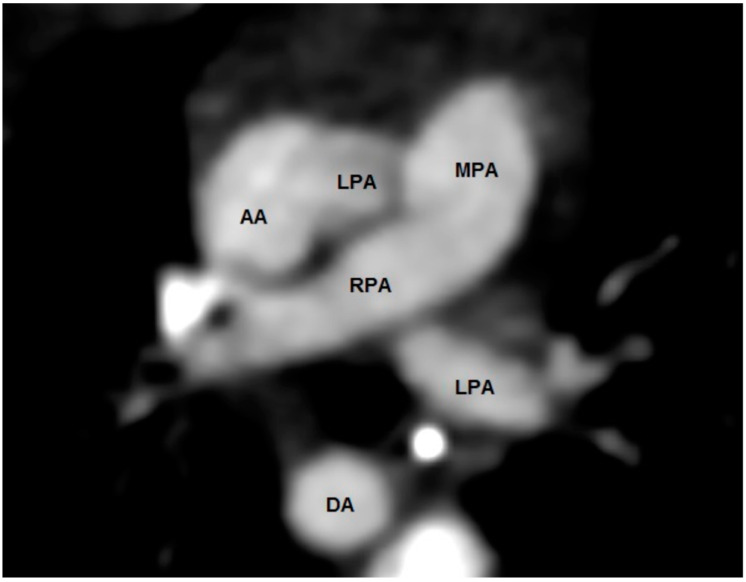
Computed tomography in the short-axis plane. The aorta is posterior and rightward to the pulmonary trunk. The pulmonary trunk gives rise to the right pulmonary artery, but not a left pulmonary artery. The left pulmonary artery arises from the anterior aspect of the ascending aorta, and then courses leftward, crossing the origin of the right pulmonary artery.
